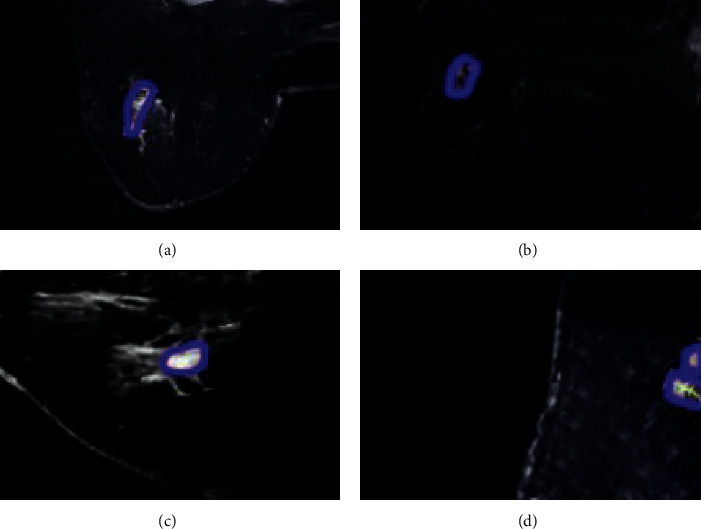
MRI images of the patient after neoadjuvant chemotherapy. (a)-(b) Horizontal images. (c)-(d) Sagittal images. Blue areas are breast masses.
